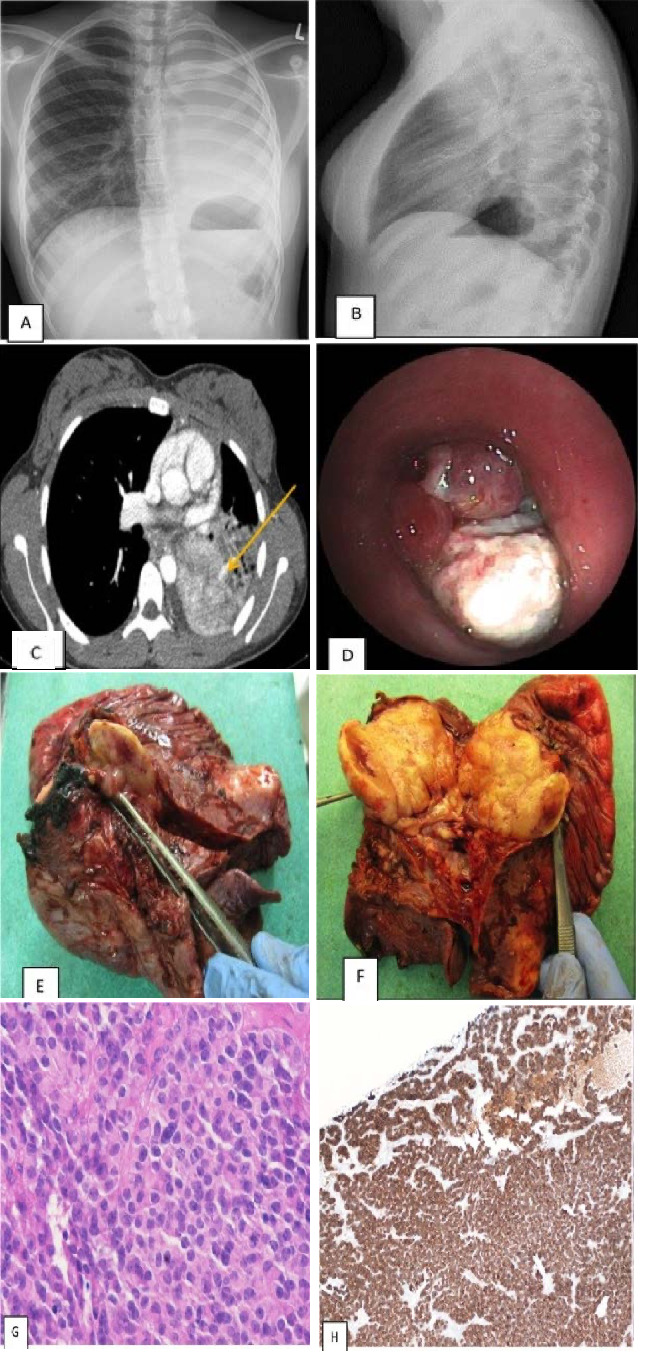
(A and B) Chest radiograph PA and lateral showing left lung
